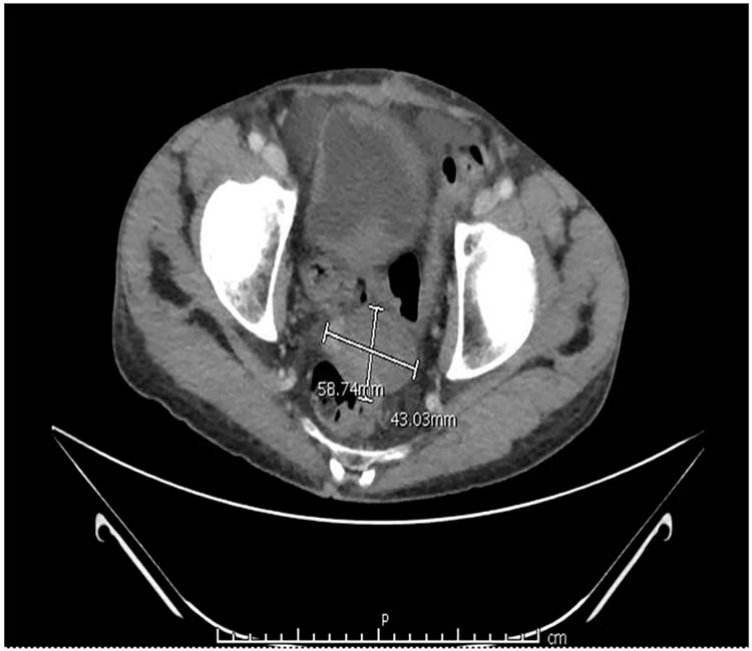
CT abdomen and pelvis with contrast identified small bowel distention and a mass-like focus measuring 5 × 3.8 cm in the pelvic region.
